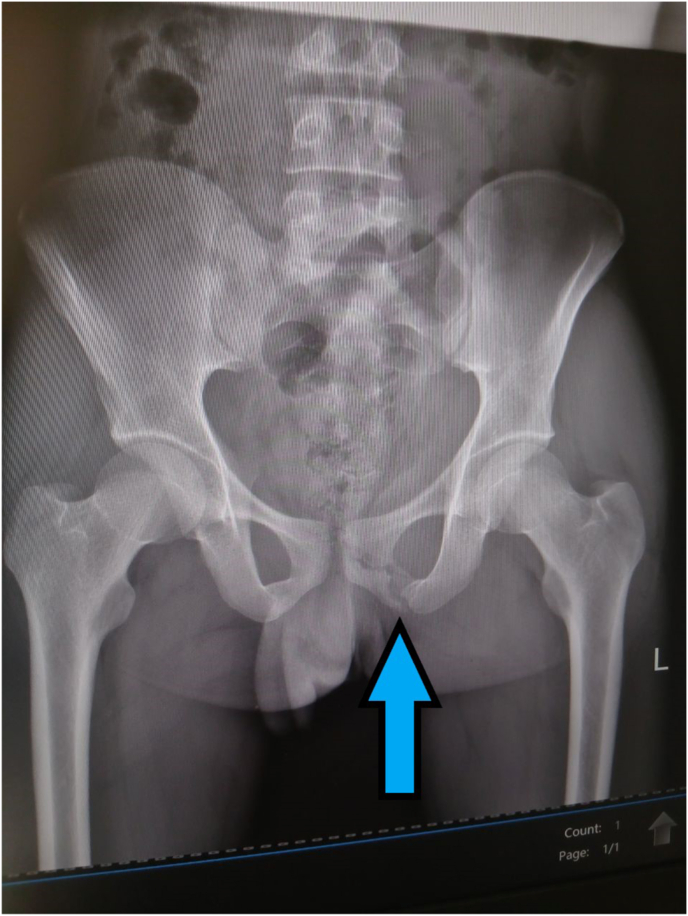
Pelvic radiography showing pelvic ramus fracture (arrow).
